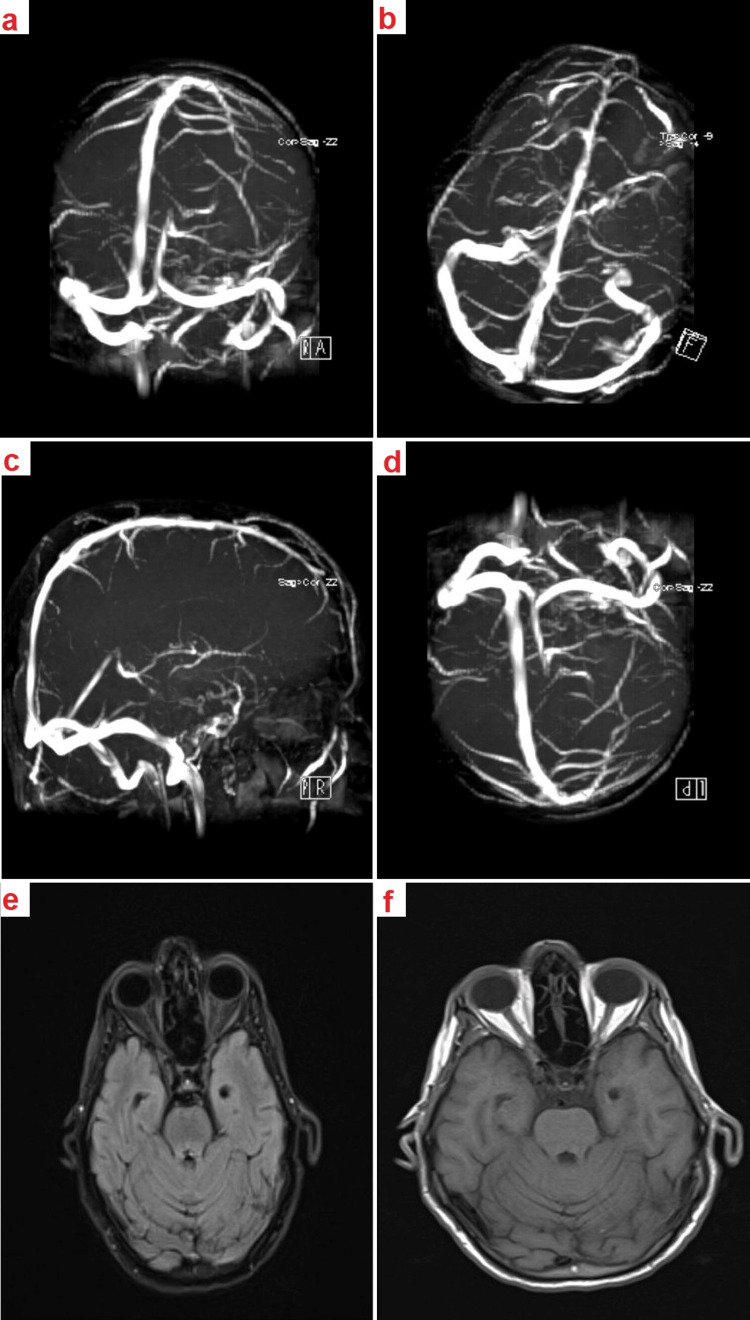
Brain Magnetic Resonance Venography (MRV) and Magnetic Resonance Imaging (MRI) a-d: Brain MRV sequences showcasing patent venous sinuses with no evidence of venous sinus thrombosis or stenosis.
